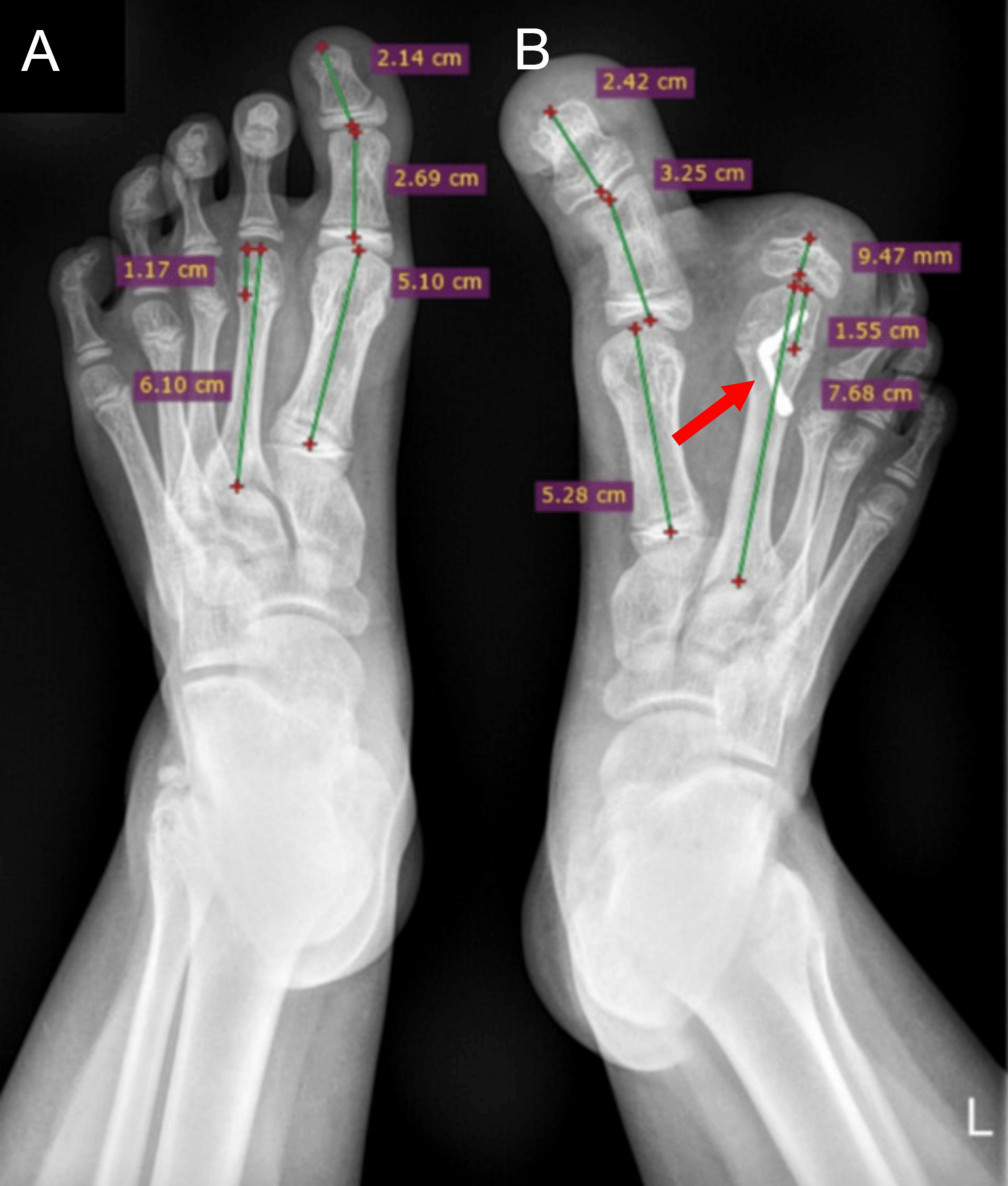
Antero-posterior (AP) X-ray of the feet at 10 years of age. Right unaffected foot of the patient (A) compared with the left macrodactyly foot (B) with visible epiphysiodesis fixing screws (red arrow).
